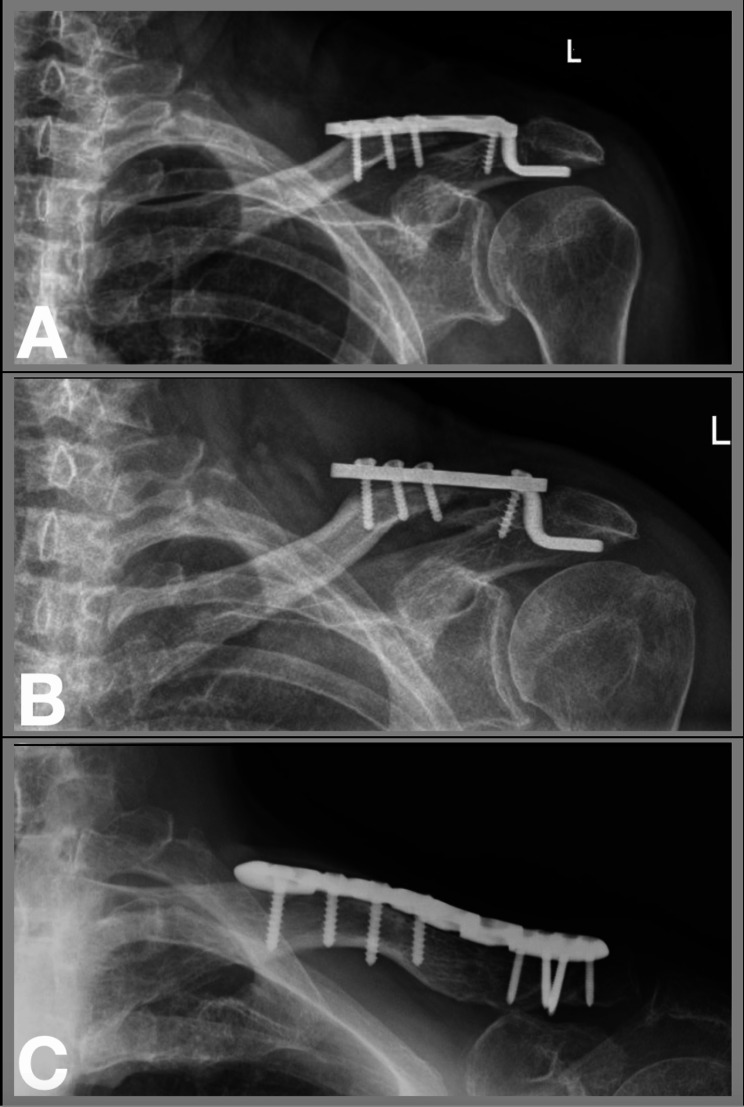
Case example of a 64-year-old female with non-union and subsequent re-osteosynthesis using an anatomical locking plate. (A) Postoperative radiograph following hook-plate, (B) Non-union and implant failure, (C) Radiograph demonstrating bone union follwoing re-osteosynthesis with an anatomical locking plate and autologous iliac crest graft
